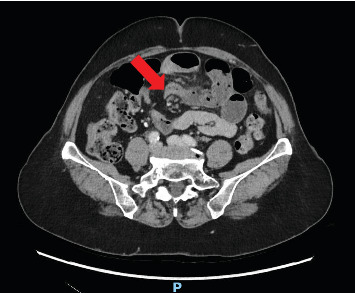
Initial computed tomography of the abdomen and pelvis with contrast showing distended bowel loops with fecalisation, indicative of a high-grade incomplete small bowel obstruction. A single transition point is noted in the lower abdomen, suggesting adhesional cause without evidence of closed-loop obstruction.
